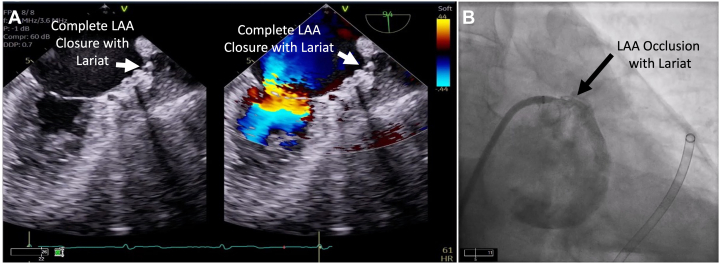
Postoperative Confirmation of Left Atrial Appendage Occlusion Complete closure of the left atrial appendage without any residual flow across the ostium was noted with transesophageal echocardiography (A) and left atrial angiography (right anterior oblique caudal view) (B). Abbreviation as in Figure 4.
